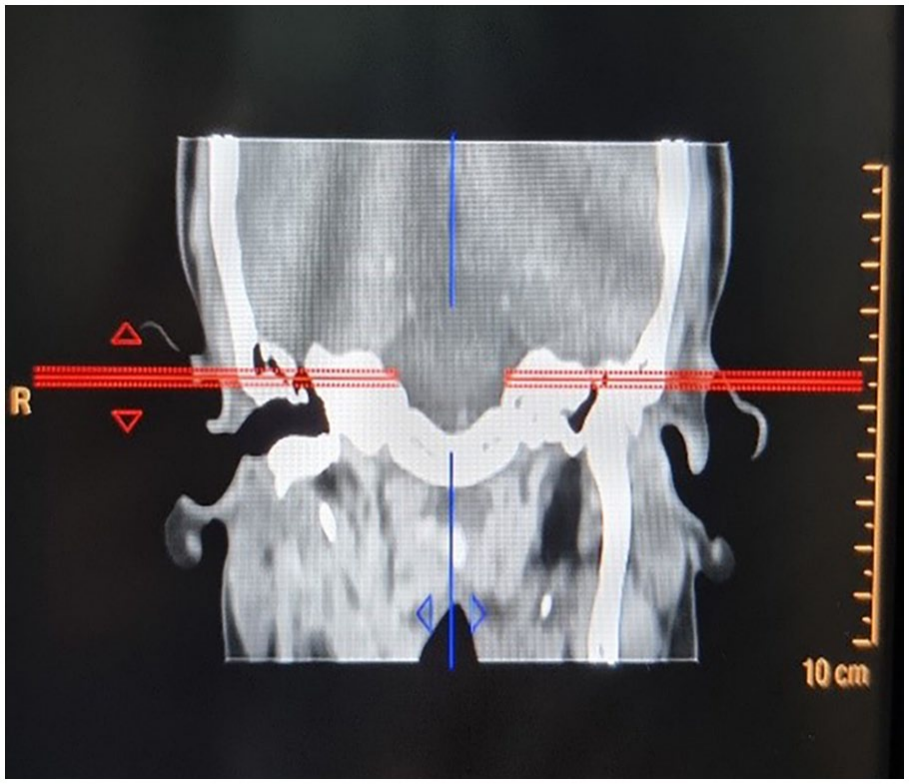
Coronal section of brain. Computed tomography (CT) image of patient’s brain.
